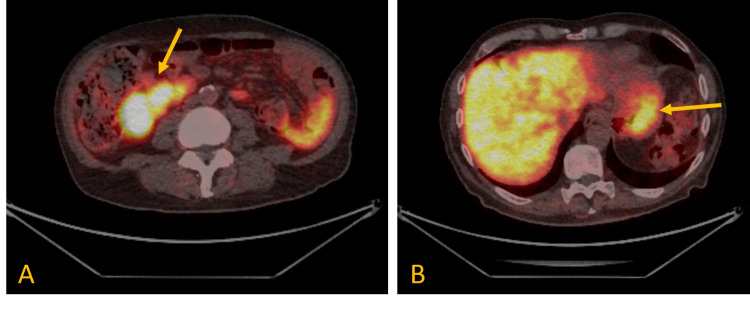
Gallium-68 DOTATATE whole-body positron emission tomography/computed tomography (⁶⁸Ga-DOTATATE PET/CT) images obtained in October 2025 Image A: Whole-body ^68^Ga-DOTATATE PET/CT image demonstrating upper abdominal adenopathy with intense DOTATATE expression (yellow arrow). Image B: Whole-body ^68^Ga-DOTATATE PET/CT image demonstrating avid uptake in the proximal stomach (yellow arrow).
